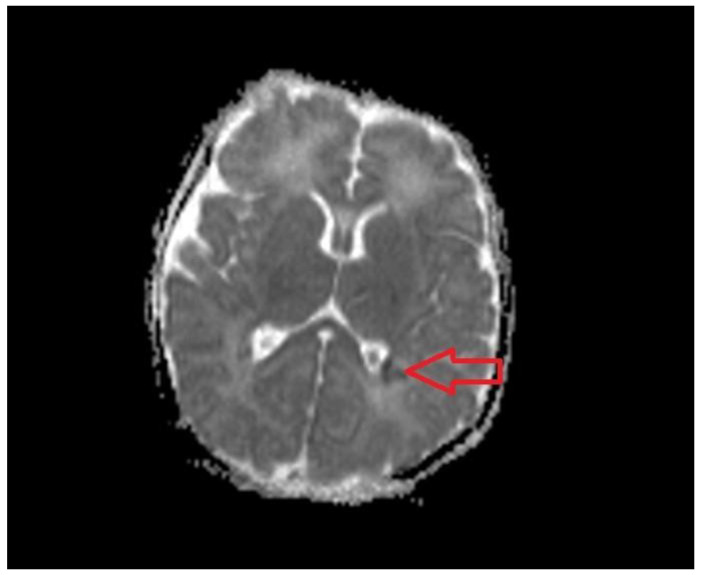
MRI on Day 5. ADC map: white matter lesion, left (shown by the arrow).
